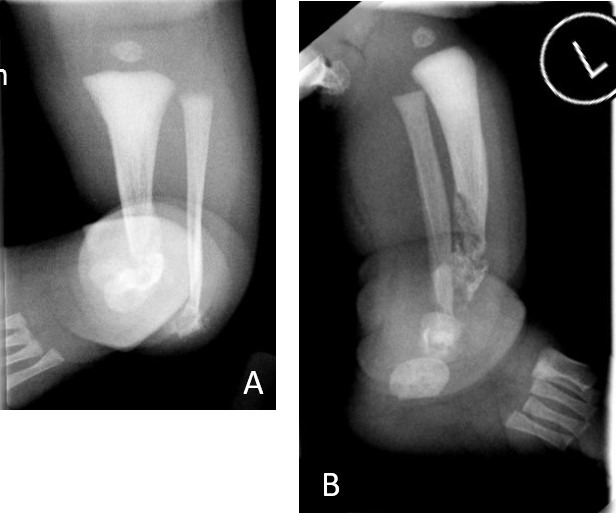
Anteroposterior (A) and lateral radiographs (B) of the left ankle at presentation (on day of birth) demonstrating mixed lytic-sclerotic lesions of the distal tibia and fibula with associated deformity
